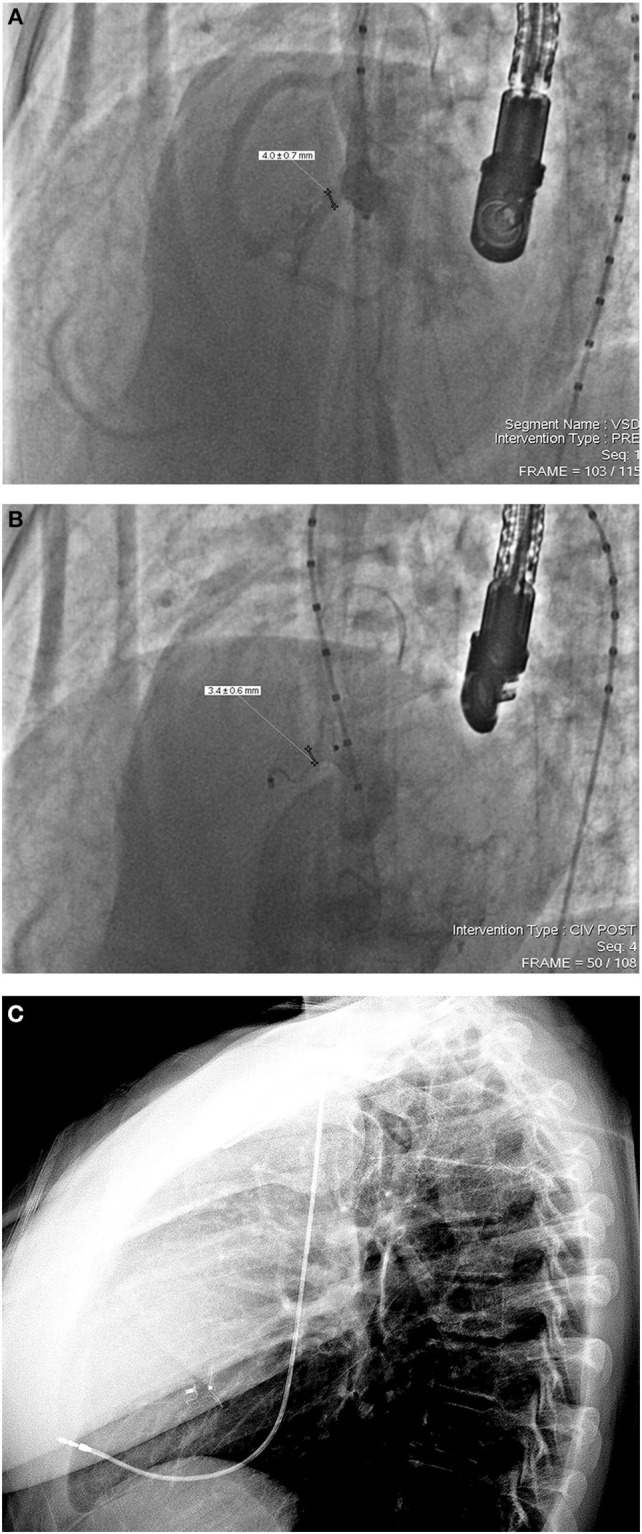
(A) Left ventricular angiography in in 55–60° left anterior oblique to 20° cranial projection incidence, showing an aneurysmal type pmVSD with a 4 mm right ventricular exist, 14 mm left ventricular entry and a 4 mm sub-aortic rim. (B) Note the compressed 12 × 10 ADO device inside the defect with a “bone-shaped” deformation immediately after release. (C) Lateral chest X-ray done the day following permanent pacing (18 months after device implantation). Note the device regaining its original conical shape despite tissue compression.
